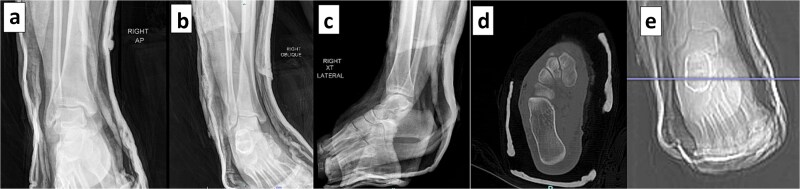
(a–c) Post-reduction radiographs obtained after bedside closed reduction and splinting demonstrate improved medial column alignment. (d–e) CT confirming adequate reduction and improved medial column alignment.
